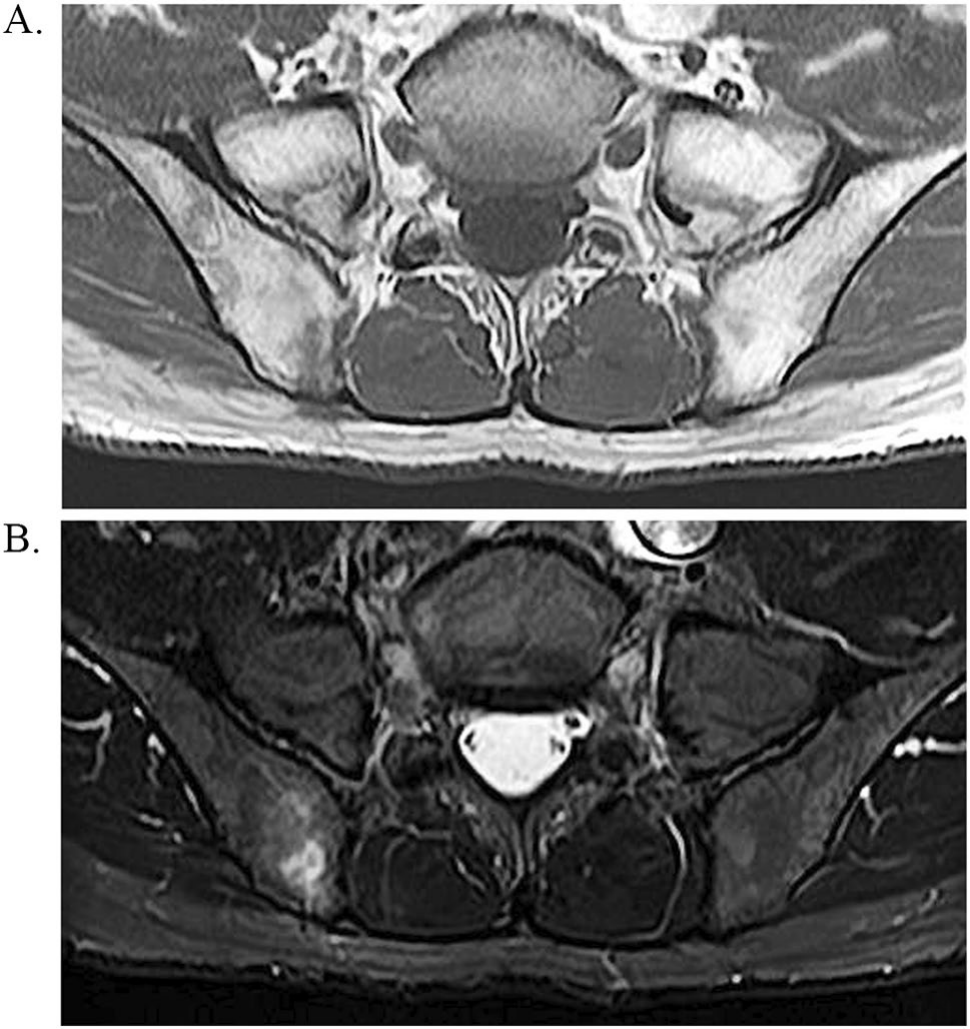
Findings of MRI on day 108. A T1-weighted images of the ilium showed diffuse high-intensity areas; however, there were focal low-intensity areas. B These low-intensity areas on the T1-weighted images showed high-intensity on the fat-suppressed T2-weighted images
